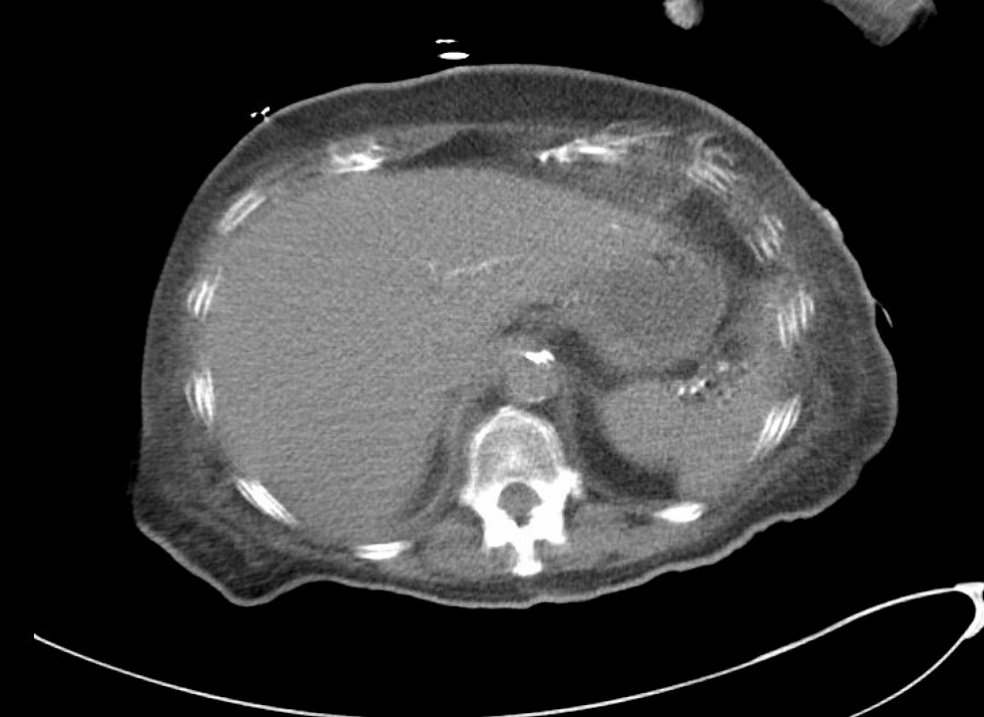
Computed tomography without contrast. The liver is noted to be within normal limits in regards to size and contour, without the presence of any focal masses.
